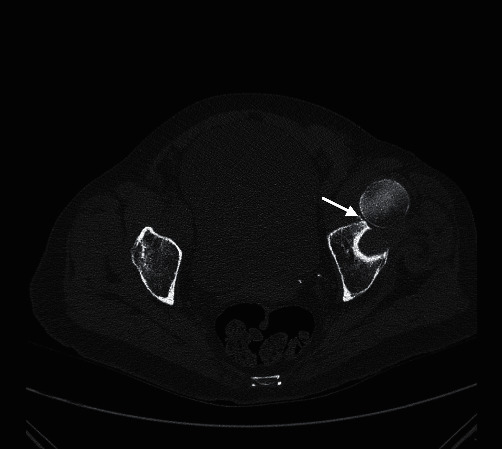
Pelvic CT scan showing recurring anterior dislocation and a minor fracture of the anterior acetabular wall [anterior capsule avulsion of the acetabulum (arrow)].
